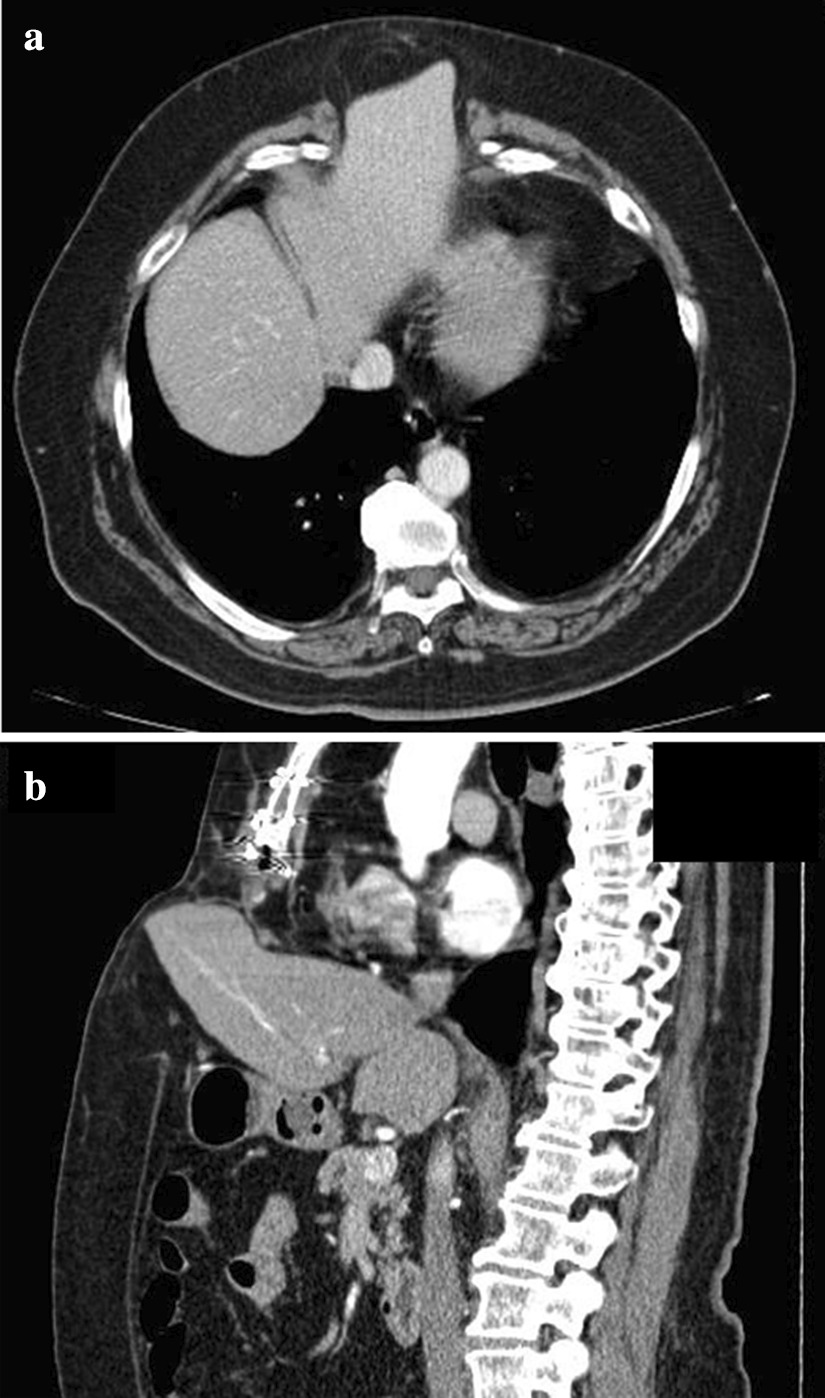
Abdominal computed tomography showing left hepatic lobe herniation through an incisional hernia. a Axial view. b Sagittal view
